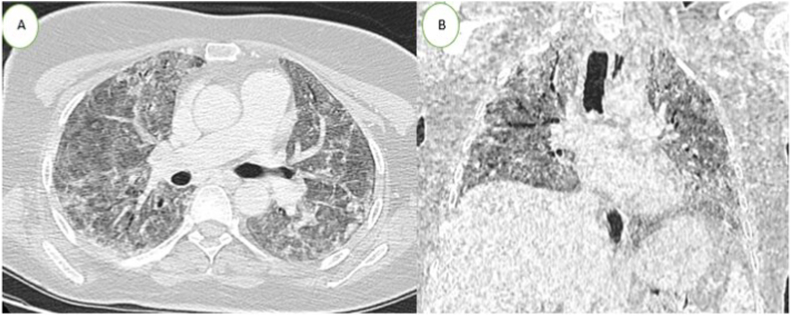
Chest CT scan in axial (A) and coronal (B) lung parenchyma windows revealing COVID-19 pneumonia related signs with more than 75% of lung parenchyma affected.
